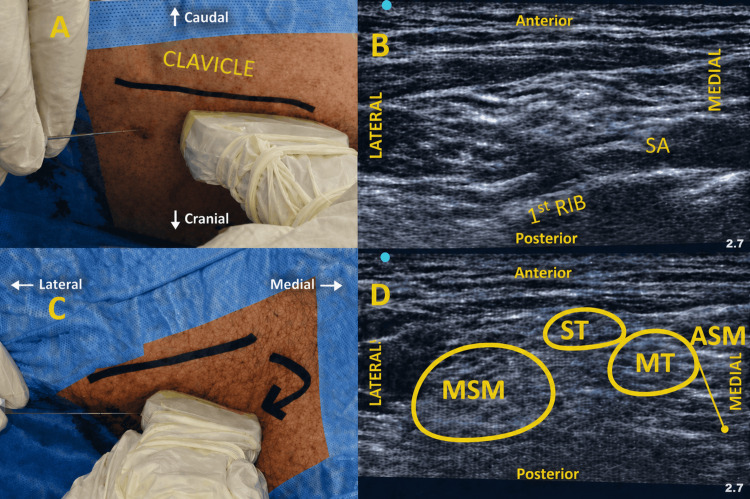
Ultrasound-guided SeTB technique (A) Patient positioned supine with the head turned contralateral to the block side. The ultrasound transducer is placed in the supraclavicular fossa to identify the inferior trunk in the corner pocket. (B) Corresponding sonographic image demonstrating the inferior trunk (IT) adjacent to the SA over the first rib. (C) Cephalad rotation of the medial end of the ultrasound transducer, without changing the lateral end or needle entry point, allows visualization of the ST and MT in the interscalene groove. (D) Corresponding sonographic image showing the ST and MT located between the ASM and the MSM. SA: subclavian artery, ASM: anterior scalene muscle, MSM: middle scalene muscle, ST: superior trunk, MT: middle trunk, IT: inferior trunk, SeTB: selective trunk block
